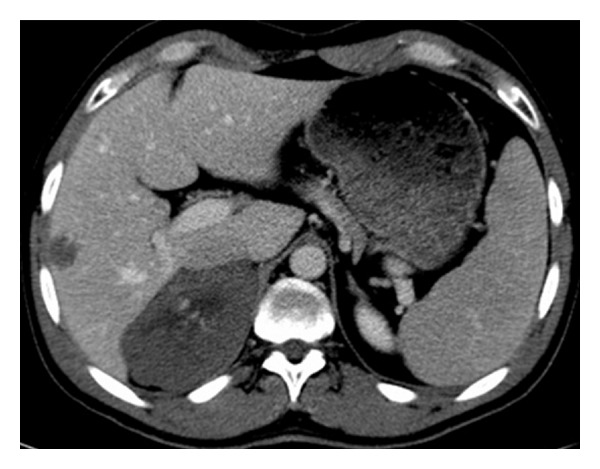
Axial postcontrast CT scan at CM phase shows a central patchy enhancement of the lesion displaying 160 HU, with nonenhancing peripheral part displaying 42 HU as well as the central hypodense area, there is a hypodense right hepatic focal lesion with nodular peripheral enhancement.
